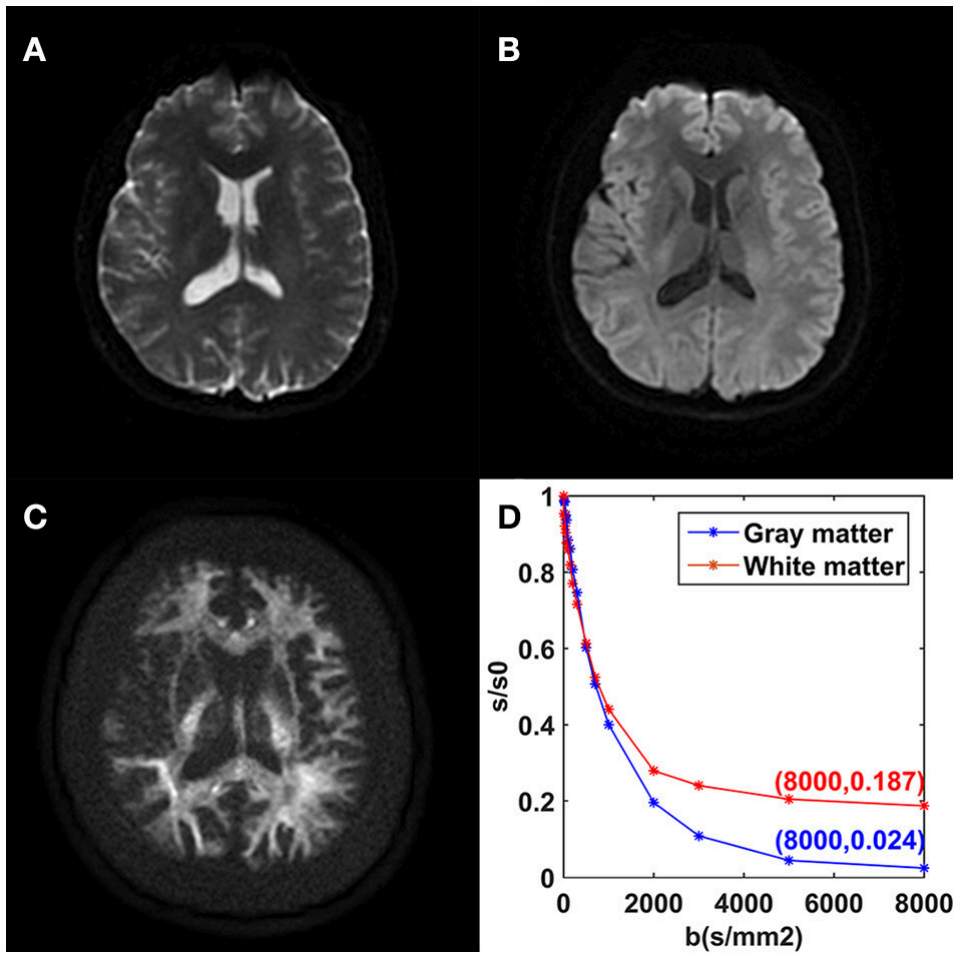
DWI images achieved with *b* = 0 (A), *b* = 1,000 (B), and *b* = 8,000 s/mm^2^
